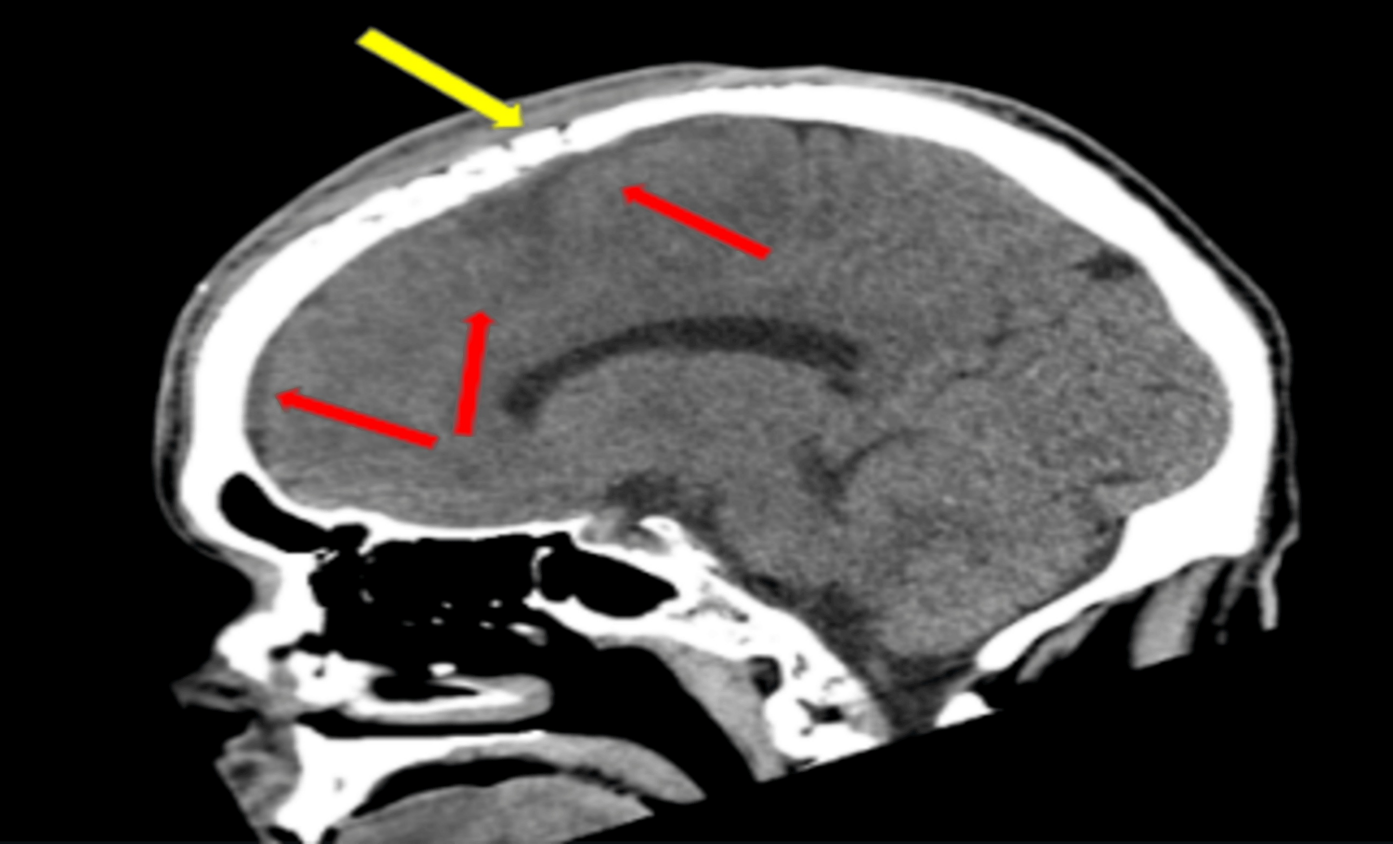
CT scan of the head. Sagittal plane of a CT scan of the head showing the subdural extension of fluid from the lytic lesion (red arrows). Erosion of the skull indicating osteomyelitis (yellow arrows).
